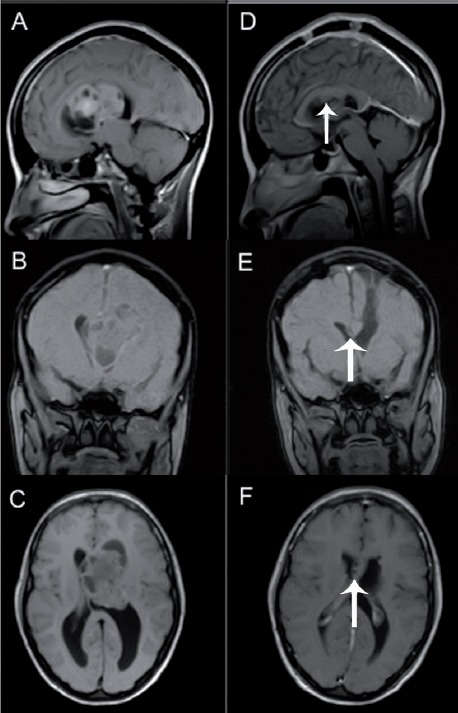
A 30-year-old woman presenting with a headache, blurry vision, and attacks of generalized body numbness for 2 months. A-C) Sagittal, coronal, and axial T1 Magnetic Resonance Imaging (MRI) with and without contrast enhancement showing third ventricular tumor with extension to the left lateral ventricle. She underwent left frontal craniotomy, frontal transcortical approach with subtotal excision of the tumor. The pathology revealed central neurocytoma. D-F) Postoperative with and without contrast enhancement T1 sagittal, coronal, and axial scans showing small residual tumor (arrow) under the corpus callosum. She postoperatively developed right side hemiparesis. In her follow-up, 6 and 9 months following surgery, her weakness improved significantly. We think the reason for her weakness is due to the retraction on the motor control areas.
